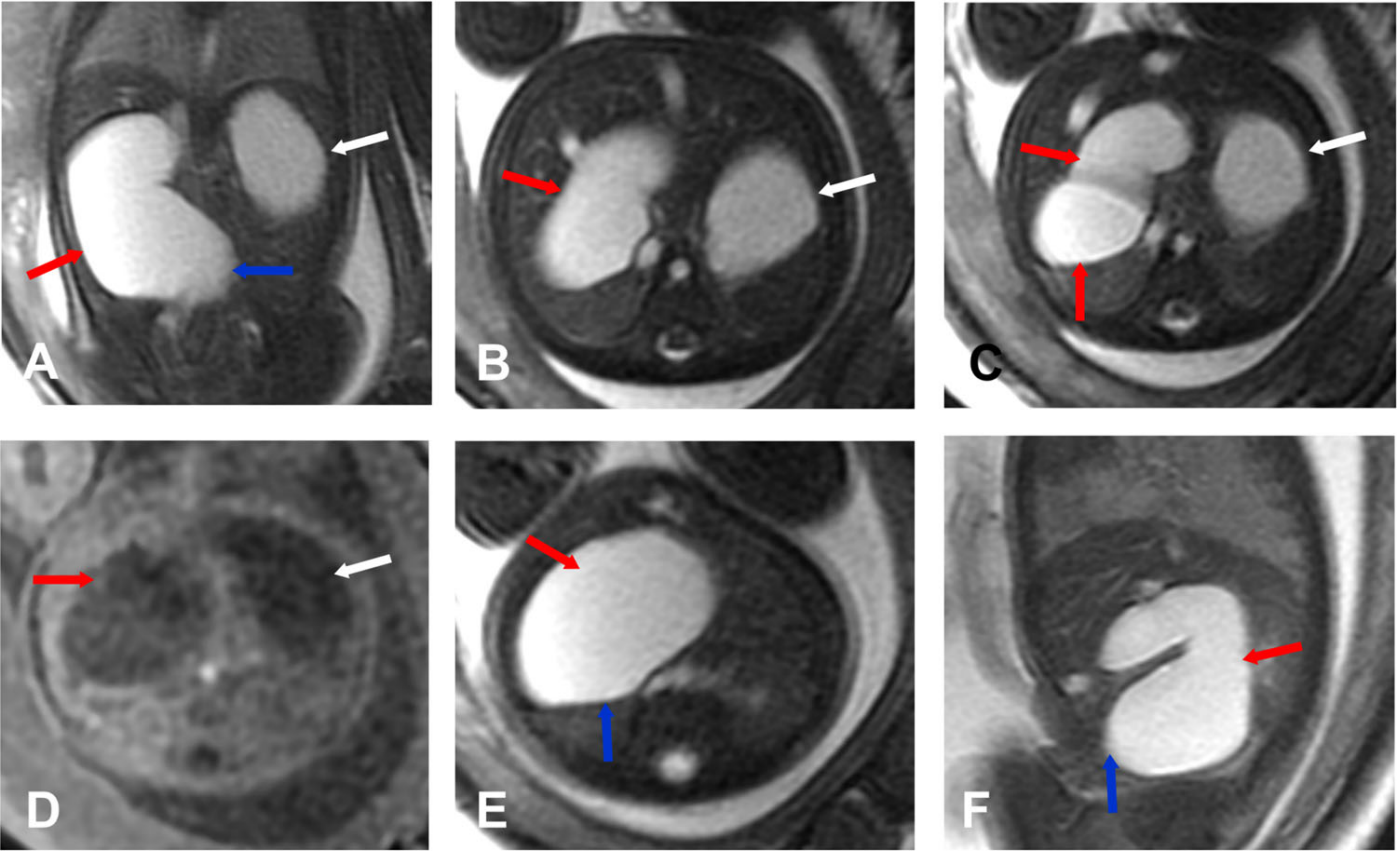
Duodenal atresia. Fetal ultrasound: duodenal atresia. Gestational age 30 weeks and 2 days. A Coronal FIESTA. B, C Axial FIESTA. D Axial 3D LAVA. E Axial FIESTA. F Sagittal FIESTA. Marked dilation of the stomach (white arrow) and duodenum (red arrow, demonstrating hypointense signal on T1 and hyperintense on T2 with the double bubble sign. Abrupt interruption of the lumen in the second duodenal bend due to atresia (blue arrow)
